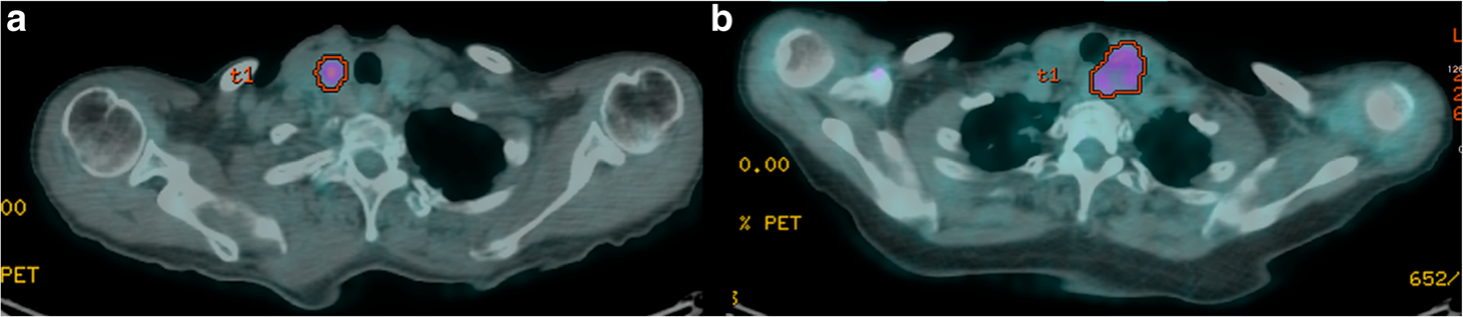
Examples of pattern of [^18^F]FDG uptake at PET/CT images of 2 thyroid incidentalomas identified by the region of interest (red circle). Moderate [^18^F]FDG uptake was observed in a benign nodule (TIR2 at cytology) of the right thyroid lobe (a) and in a differentiated thyroid cancer (TIR3 at cytology) of the left thyroid lobe (b)
